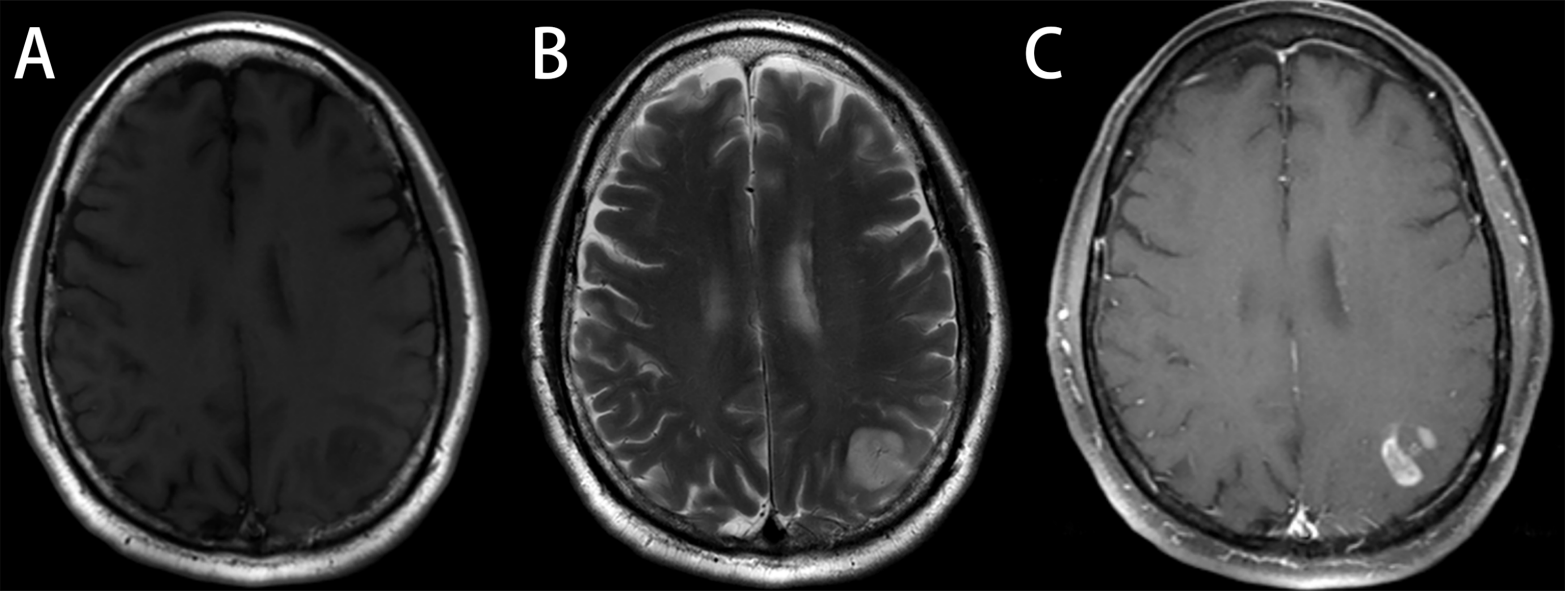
Astrocytoma, grade 3, in a 48-year-old man with a license named TCGA-CS-6188 (TCGA-CS-6188). (A) Axial T1-weighted MR images demonstrate an area of heterogeneous low signal intensity in the left occipital region with adjacent edema. (B) Axial T2-weighted MR image reveals a heterogeneous high signal in the lesion. (C) Axial T1-weighted MR image with contrast material showed significant “flower lace” enhancement in the lesion. Reader 1 diagnosed the lesion as glioma, grade 3, and the diagnosis of reader 2 and reader 3 was glioblastoma (GBM). Radiomics predict_proba_GBM was 0.512 (cutoff value = 0.610), and the radiomics model diagnosed it as low-grade glioma (LGG).
